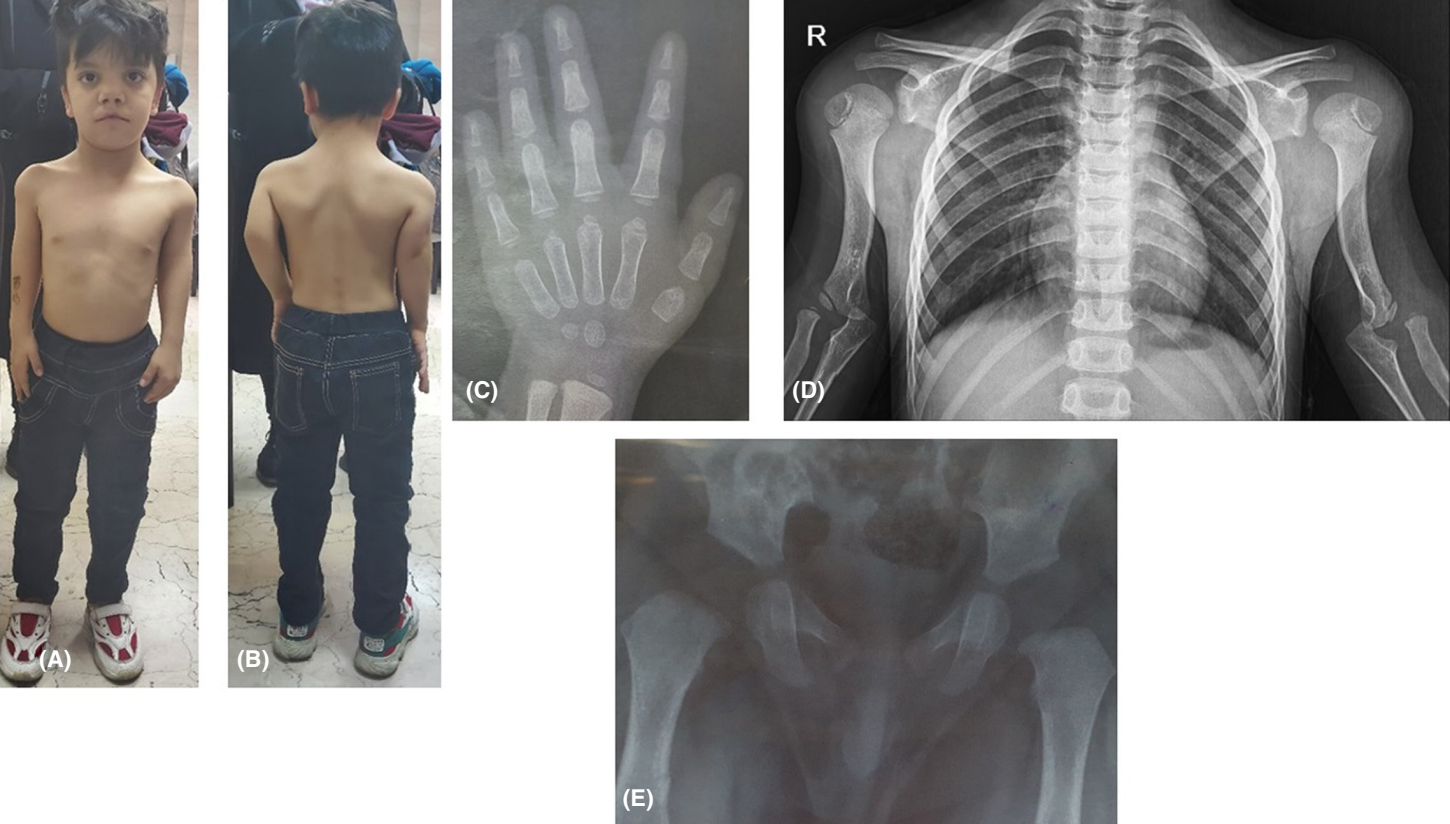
Characteristics of patient. Short humerus, dysmorphic facial features including a large forehead, round face, hypertelorism, depressed nasal bridge with short nose and a long philtrum, cleft lip, and anteverted Nares. The height of patient was normal (A), limited extension in left elbow (B), short first metacarpal on right hand radiography (C), Bilateral radial head dislocation and proximal radioulnar joint disatasis in chest X‐ray (D). Normal hip radiography (E). The C and E radiographies are for when the patient was 4‐year‐old
